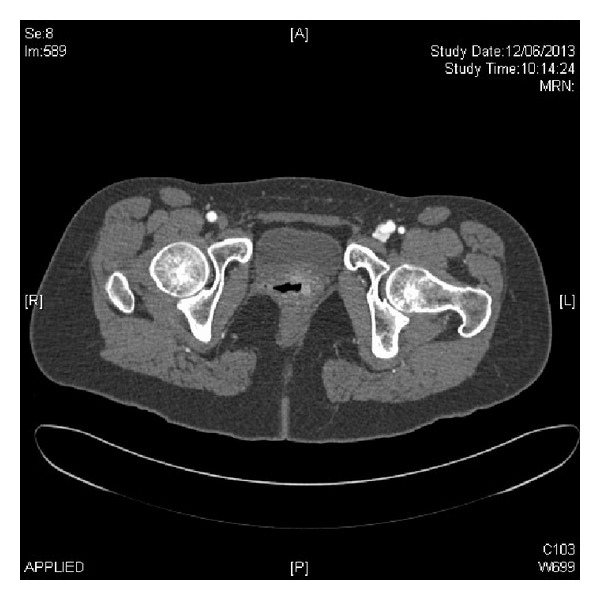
A CT angiogram demonstrating the abnormal contrast flow between the SFA and CFV, diagnosing the arteriovenous fistula.
